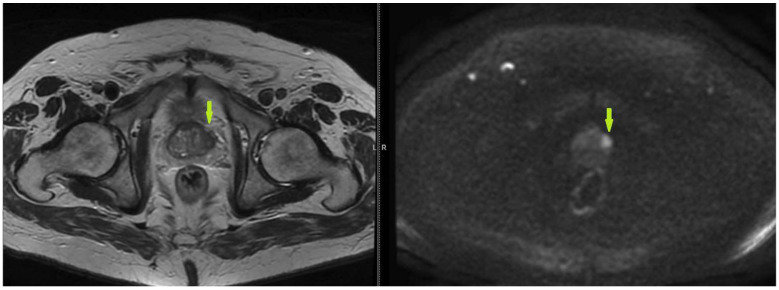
T2 and DWI sequences of a prostate MRI, showing a lenticular lesion, uncircumscribed, with T2 hyposignal and restricted diffusion, localized in the transitional left zone, with peripheric extension—scored PI-RADS 5 (green arrow).
